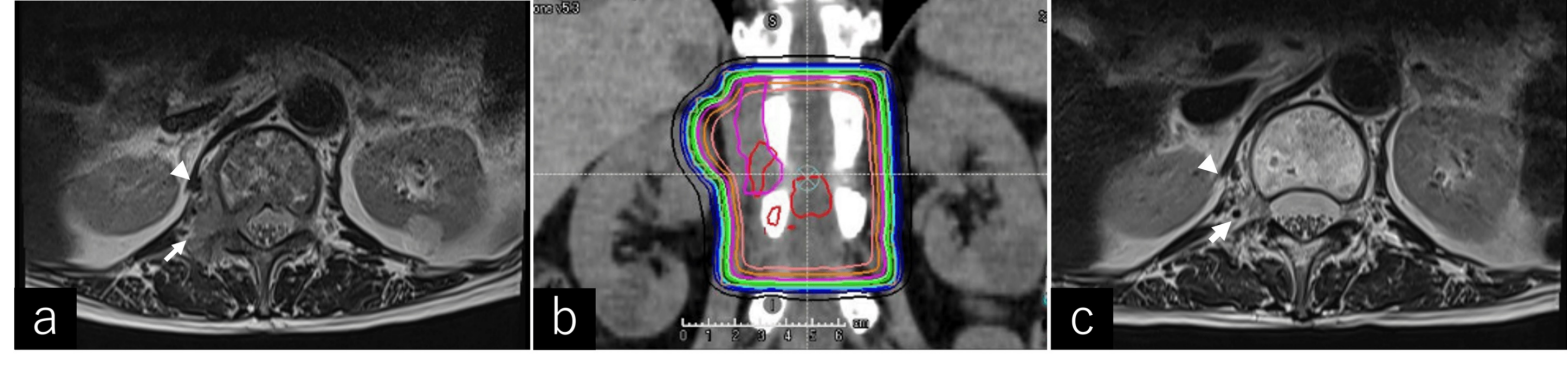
Case 2: MRI and radiation therapy planning for lumbar spine tumor invasion. Magnetic resonance imaging of the lumbar spine revealed tumor invasion of the intervertebral foramen of the 1st lumbar vertebra (arrow), with no evidence of significant invasion into the psoas muscle (arrowhead) (a). RT planning involved irradiation from two fields in the anterior-posterior direction. The patient received 37.5 Gy in 15 fractions of RT for the 1st and 2nd lumbar vertebrae (b). A T2-weighted image obtained 1 month after radiation therapy showed a reduction in tumor size (arrow and arrowhead) (c).
